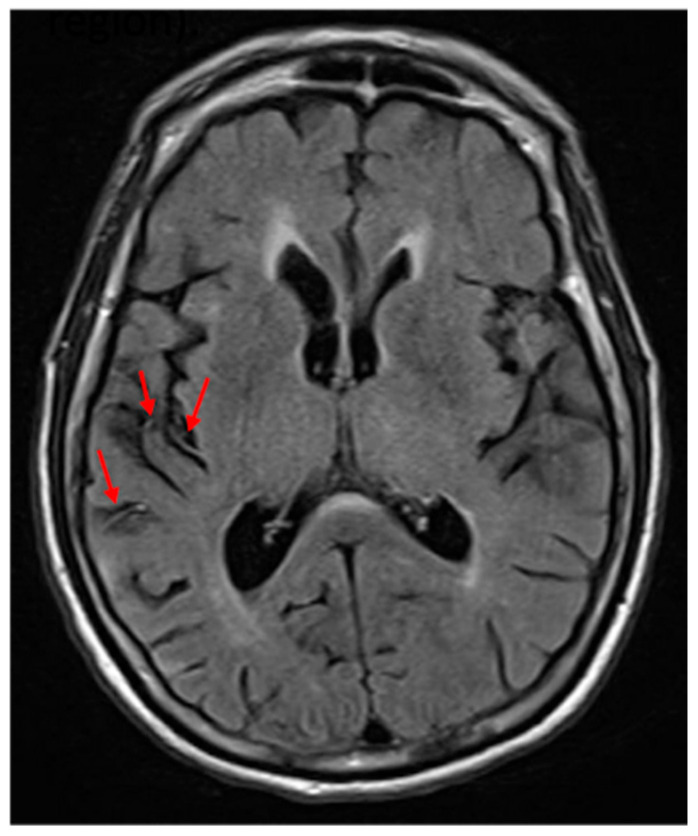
FLAIR hyperintense vessels (two in the middle cerebral artery frontal region and one in the insular region). The red arrows point to the FLAIR hyperintense vessels.
